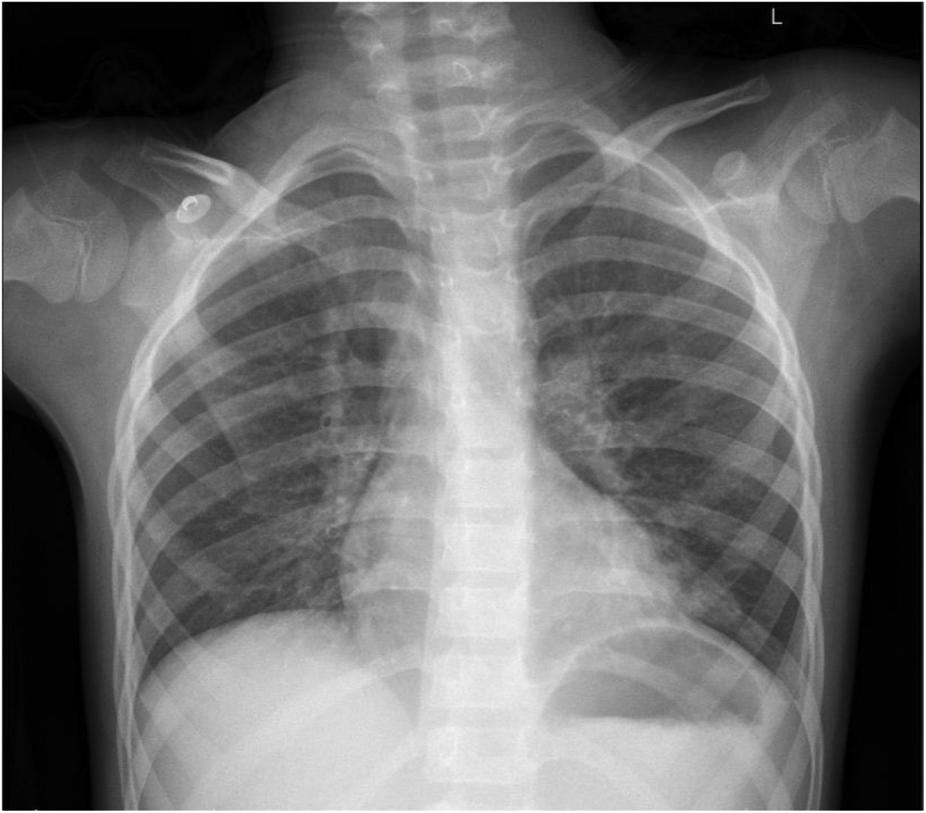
Reviewed chest x-ray before discharge (the lesion in the left lung has been mostly absorbed).
